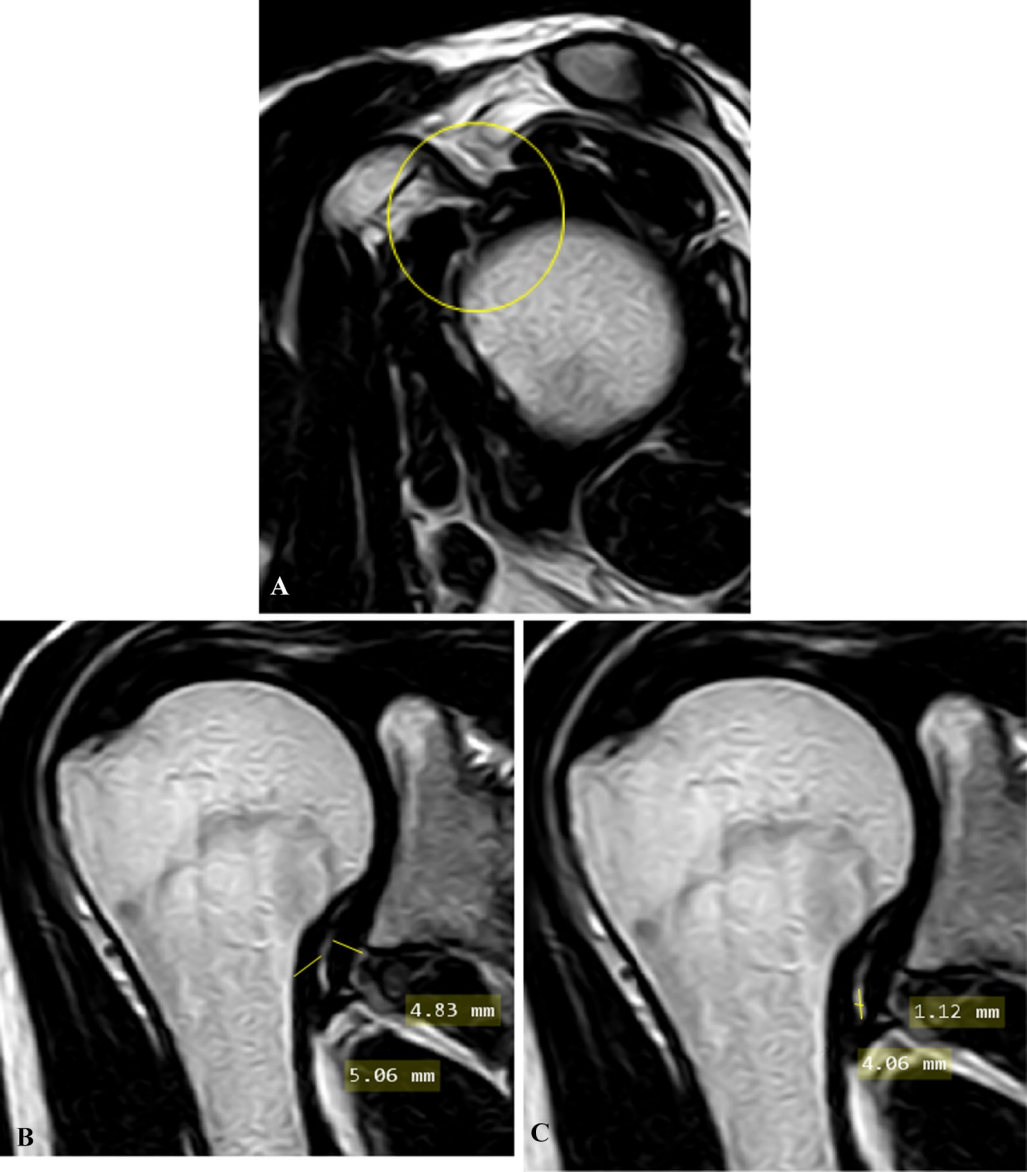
A Oblique sagittal T2-weihted MR image shows preserved fat planes and coracohumeral ligament of the rotator interval (encircled area). B Oblique coronal T2-weighted MR image shows thickened joint capsule and measurement of maximum capsular thickness of glenoid and humeral portions of axillary recess. C Oblique coronal T2-weighted MR image shows measurement of maximum height and width of axillary recess at the same plan, it was fibrotic and obliterated
